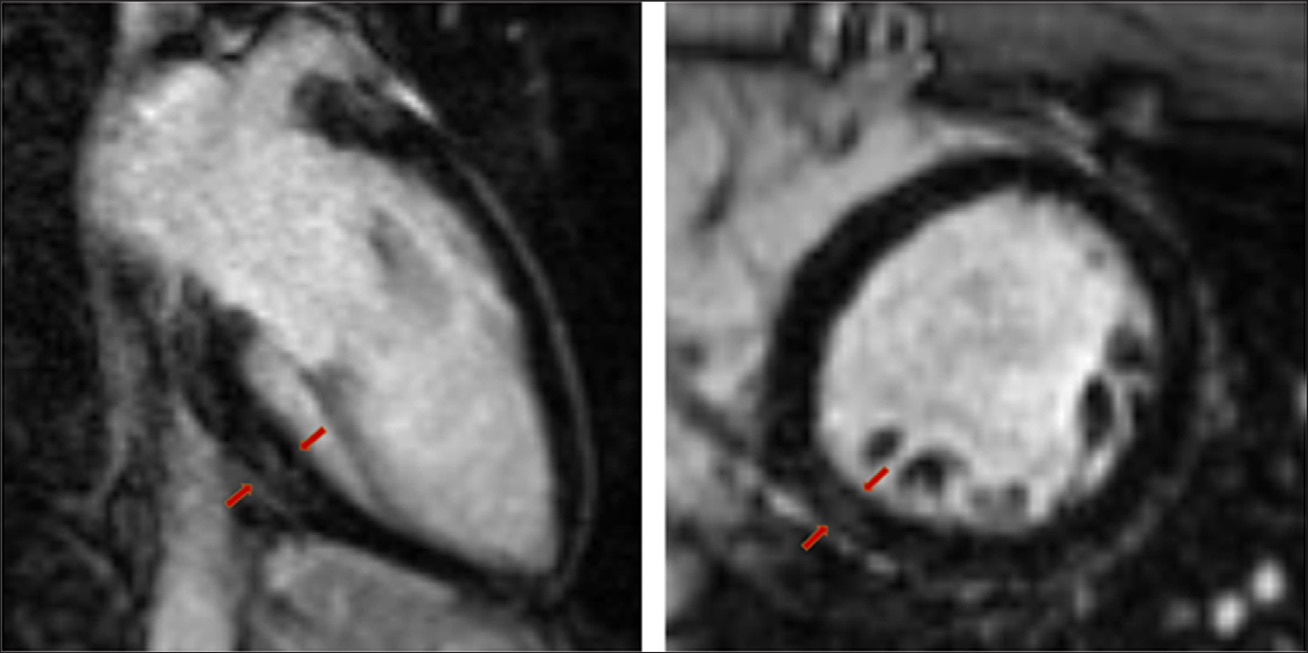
Cardiac magnetic resonance image of 25-year-old healthy male complaining of persistent chest pain and palpitations 5 weeks after COVID-19 diagnosis. Mild late-gadolinium enhancement can be visualized in the basal inferior and inferolateral myocardial wall. Holter monitoring showed frequent premature ventricular beats.
